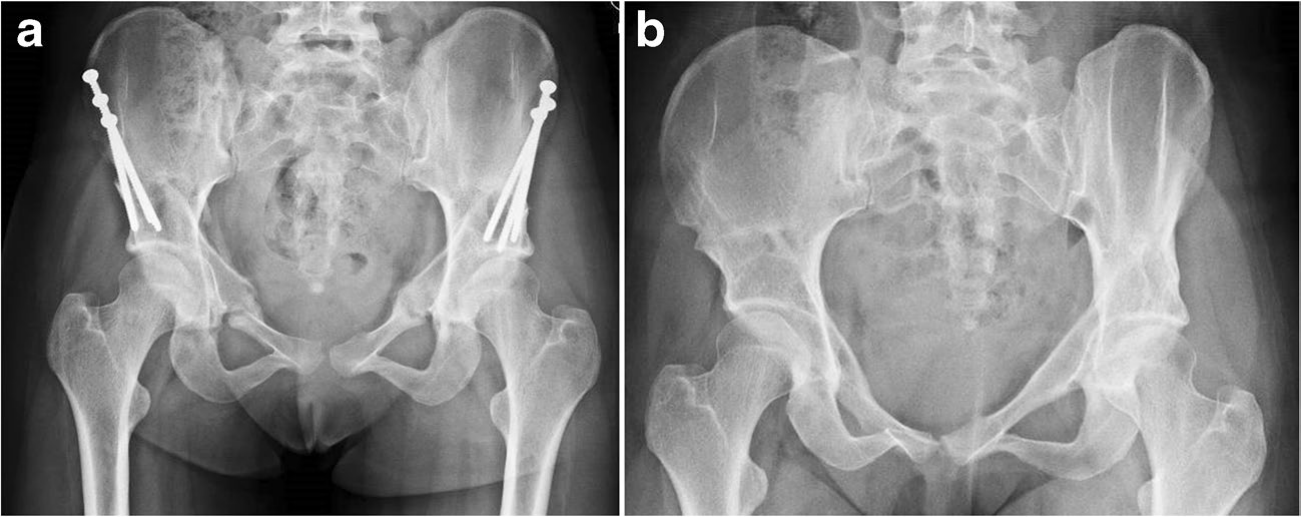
Post-operative imaging: a plain AP view radiograph after periacetabular osteotomy: a with screws in situ and b fully healed osteotomy following screw removal
